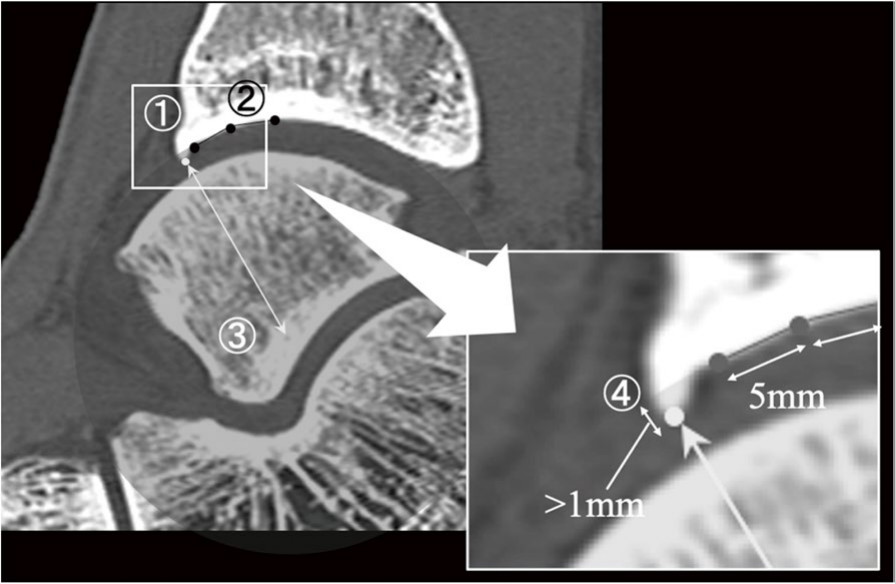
First, we marked a dot on the anterior tip of the distal tibia's articular surface on the sagittal CT view using freeware Image J. Second, we marked two more dots at 5 mm intervals from the first dot on the articular surface. Third, we calculated the coordinate of the center of a circle passing through these three dots, which correspond to the morphology of the anterior part of the tibial articular surface. Lastly, we marked a dot on the osteophyte tip and calculated the distance between the circle’s center and the dot on the osteophyte tips from their coordinates. If the distance is shorter than the circle’s radius by > 1 mm, osteophyte protrusion is confirm to be present
